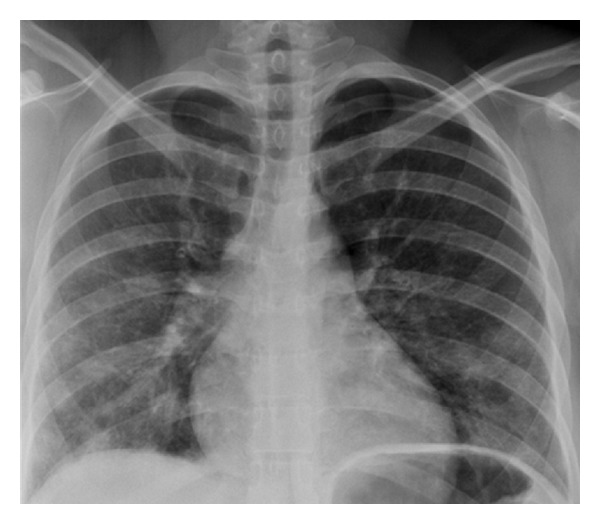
A 30-year-old female after illicit silicone injection. Findings: PA chest radiograph demonstrates diffuse haziness throughout the middle and lower lungs especially in the right lower lung.
